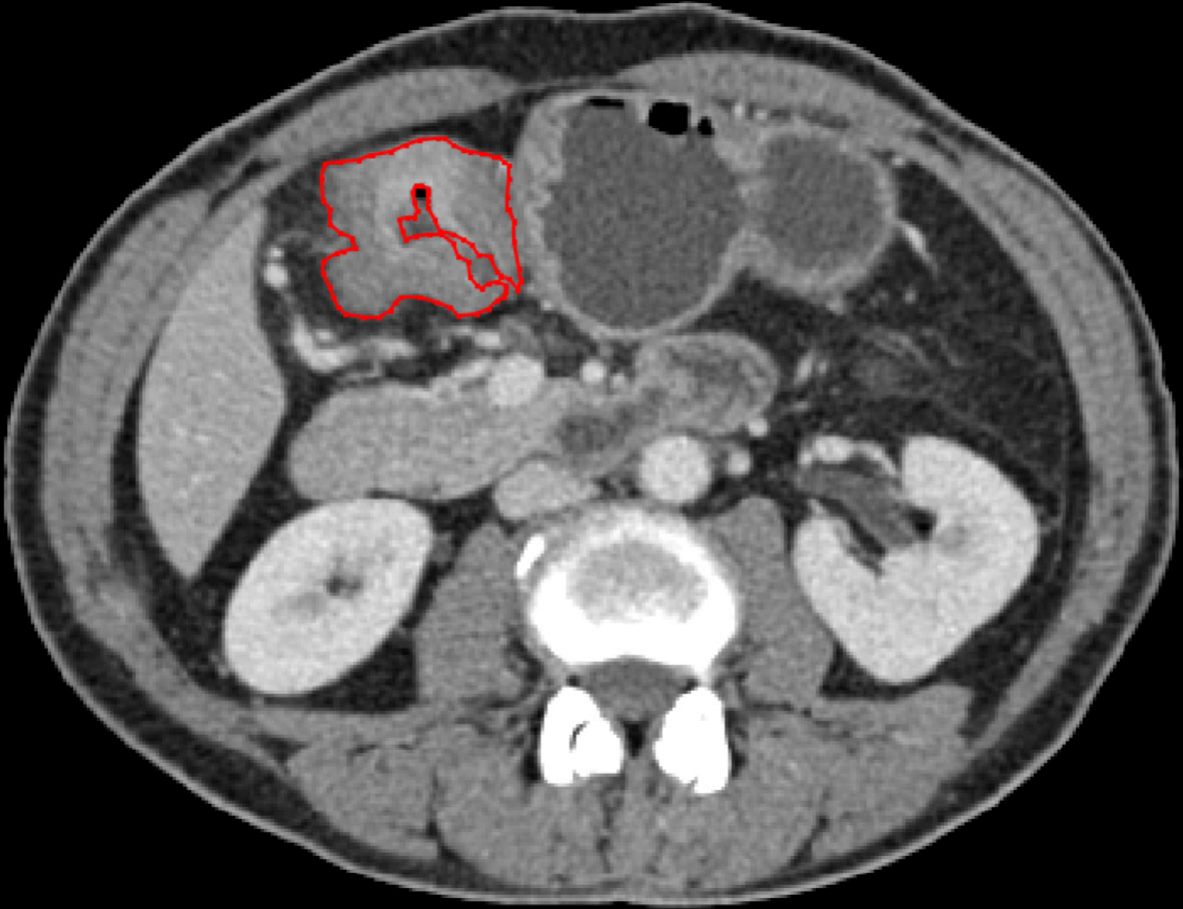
ROI annotation (red line) on a representative CT image, with the largest area of the primary lesion drawn on the axial plane.
